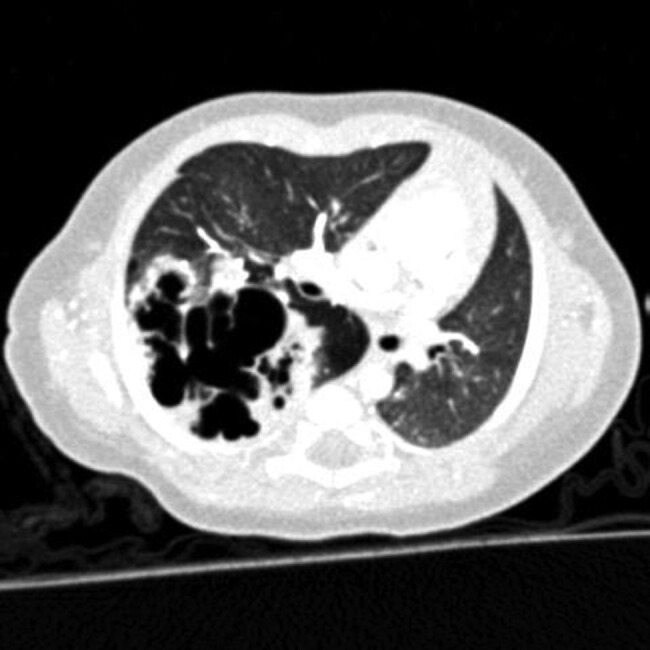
Transverse CT image showing a right lower lobar congenital pulmonary airway malformation (CPAM)
